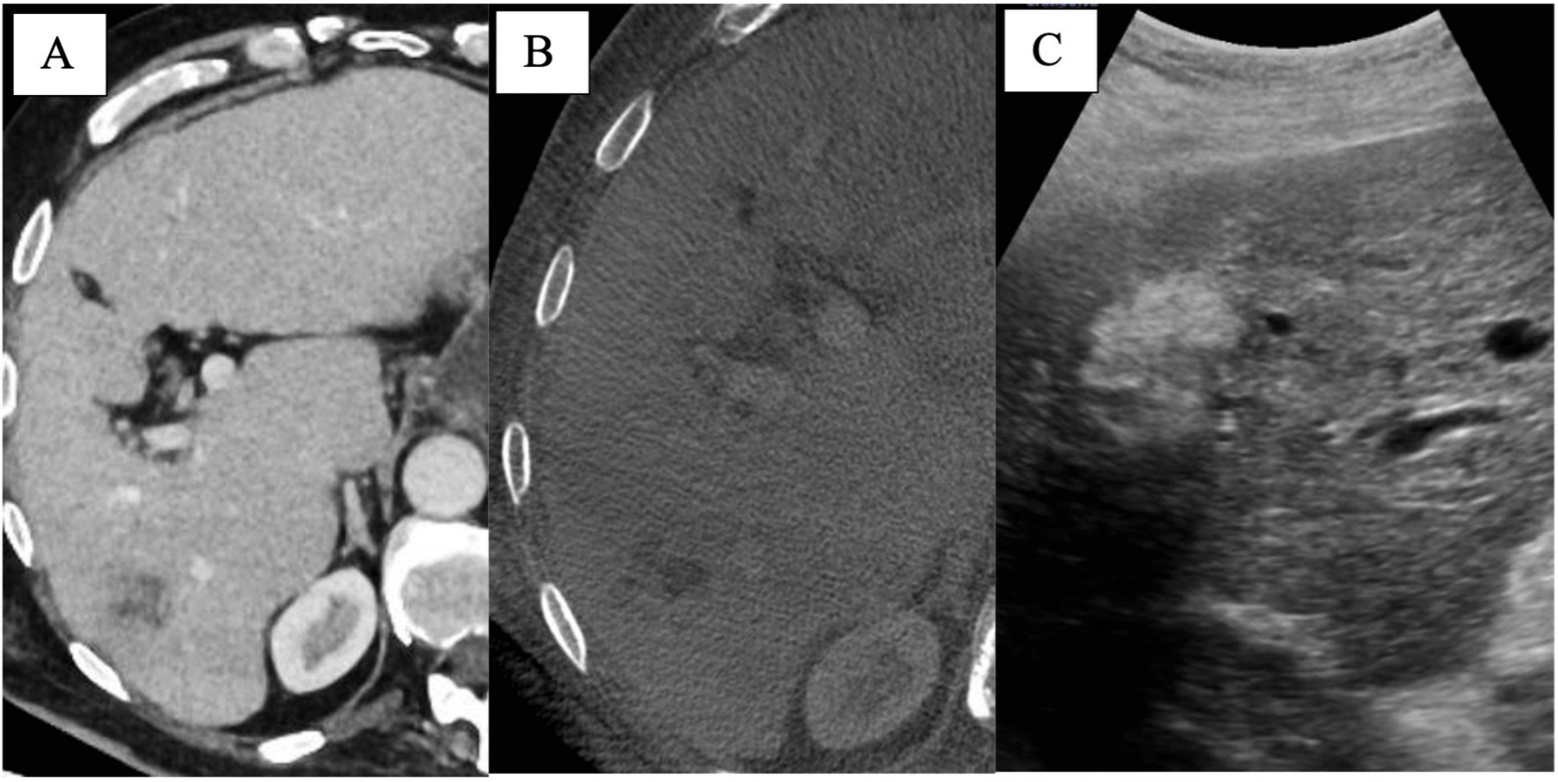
MWA of primary HCC: lesion appearance prior to ablation A single HCC lesion is shown on conventional CT prior to ablation (A). Its appearance on angiographic Dyna-CT is shown in image (B) as well as its appearance on ultrasound prior to probe placement (C). MWA: microwave ablation; HCC: hepatocellular carcinoma; CT: computed tomography; Dyna-CT: dynamic volume computed tomography
